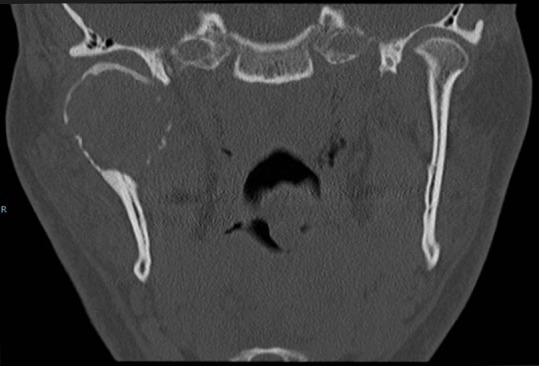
Coronal CT (bone window) depicts expansion of the right mandibular condyle by a soft tissue cancellous lesion with partial interruption of cortical bone and marked shortening of ascending ramus. Note lack of intraosseous trabeculae or calcification.
